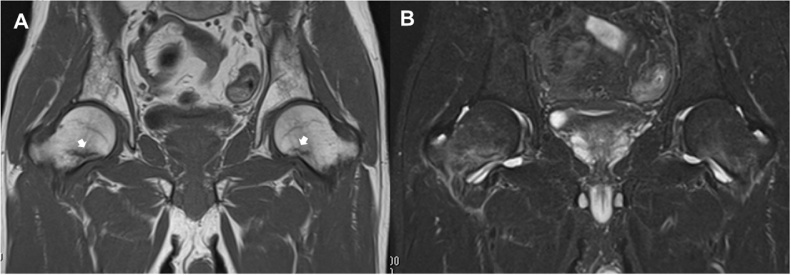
(A) T1 weighted MRI showing macroscopic fracture (white arrows). Fracture measures ≥50% of femoral neck width in coronal plane on right hip and <50% of femoral neck width in coronal plane on left hip. (B) MRI on short τ inversion recovery (STIR) sequence showing clear bone marrow edema in right femoral neck and slight bone marrow edema in left femoral neck.
